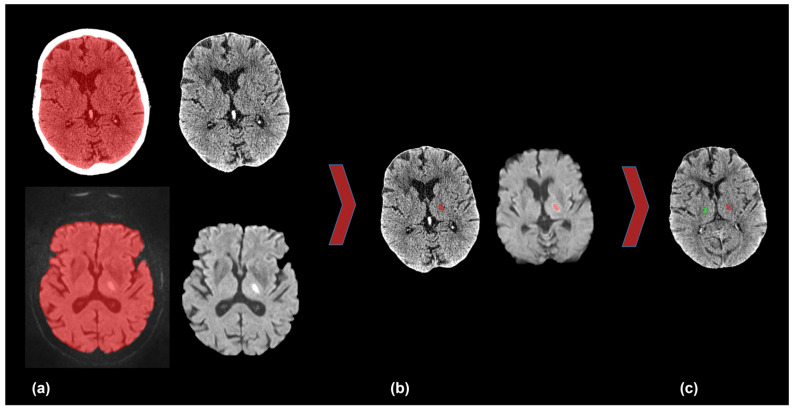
Co-registration of DW-MRI and NCCT. (a) Semi-automatic segmentation and brain extraction in NCCT (upper row) and DW-MRI (lower row). (b) Co-registration of NCCT and DW-MRI and infarcted area in the left thalamus (red). (c) Mirroring the left-sided infarct (red) onto the unaffected contralateral right thalamus (green). DW-MRI = diffusion-weighted magnetic resonance imaging, NCCT = non-contrast computed tomography.
